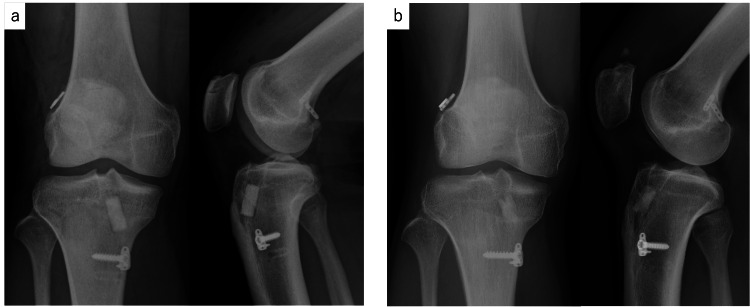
Antero-posterior and lateral views of radiographs at (a) immediate postoperative and (b) one year postoperative for case 7
